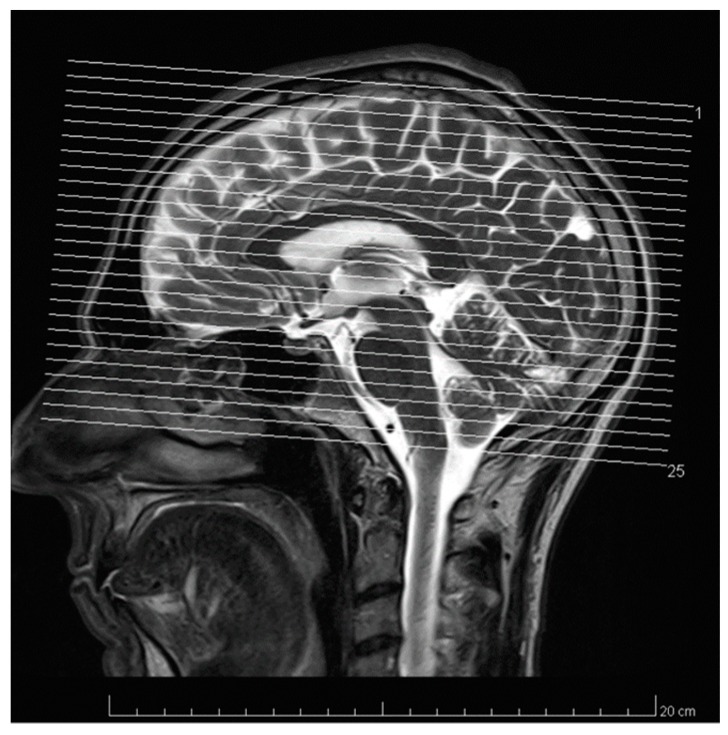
Magnetic resonance tomography (MRT) cut picture of a human head as an example for the MRI possibilities. From: Private.
